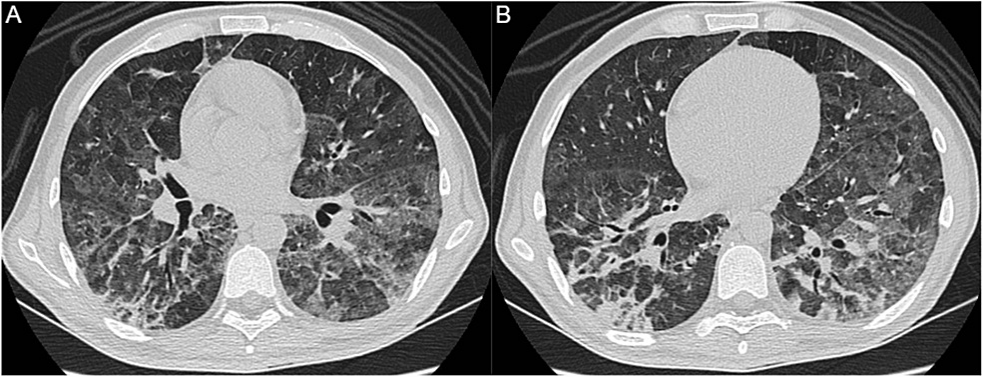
CT of the chest showing (A) ground-glass opacities in both lungs and (B) consolidation in both lower lobes CT: computed tomography
